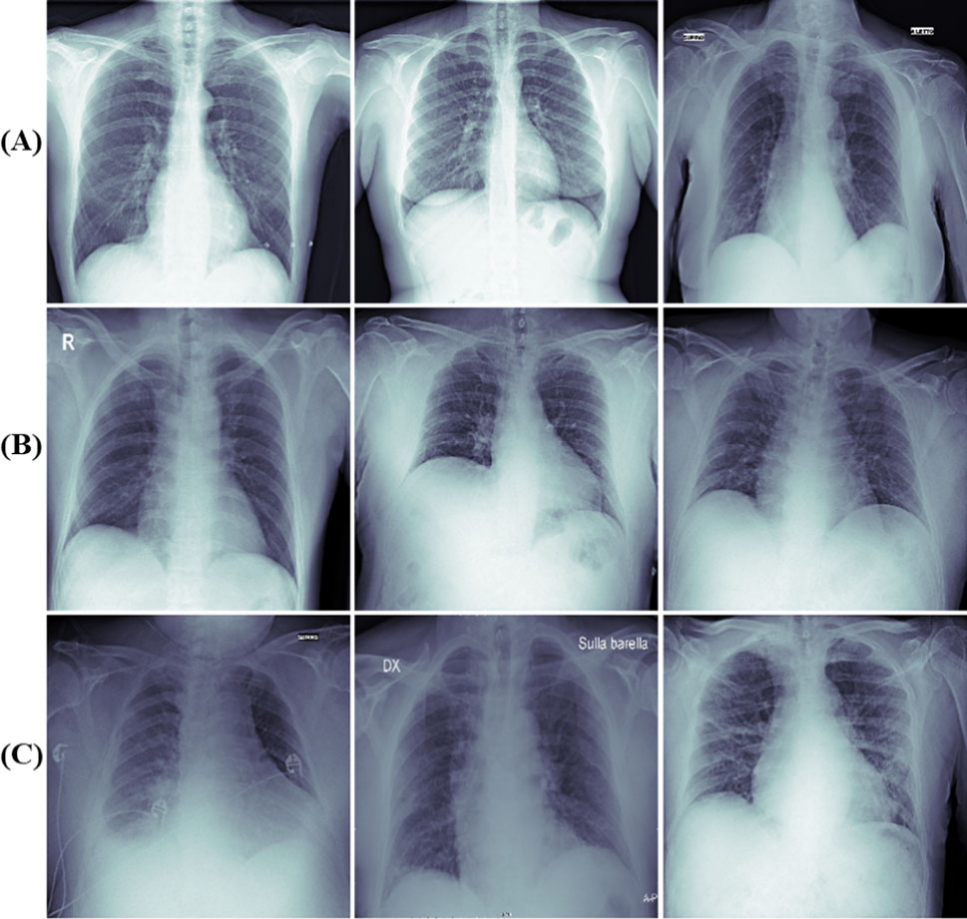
Chest X-ray sample images for COVID-19. A Low-risk patients, B High-risk patients with survival outcomes, and C High-risk patients with death outcomes
